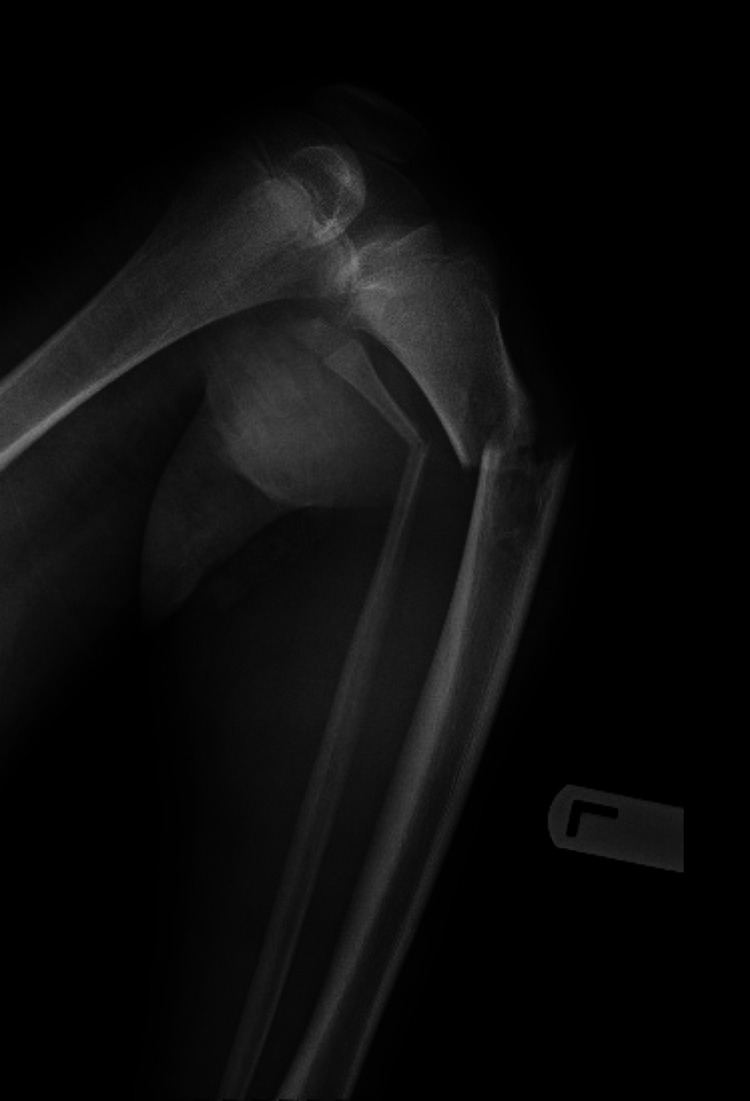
X-ray of the left leg on initial presentation
